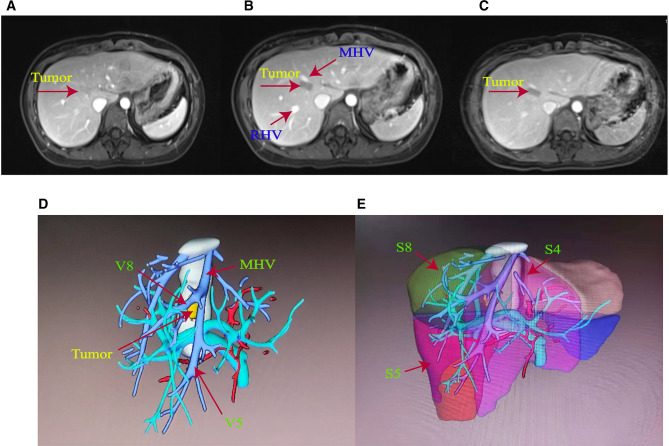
(A) Preoperative MRI of the patient with enhanced arterial phase. (B) Preoperative MRI of the patient with enhanced venous phase. (C) Delayed phase of preoperative MRI enhancement in the patient. (D) Main hepatic vein (MHV) trunk and V5 and V8 branches were labeled in accordance with the three-dimensional stereo images of hepatic vessels reconstructed by imaging. (E) S4, S5, and S8 reconstruction images of liver segments.
